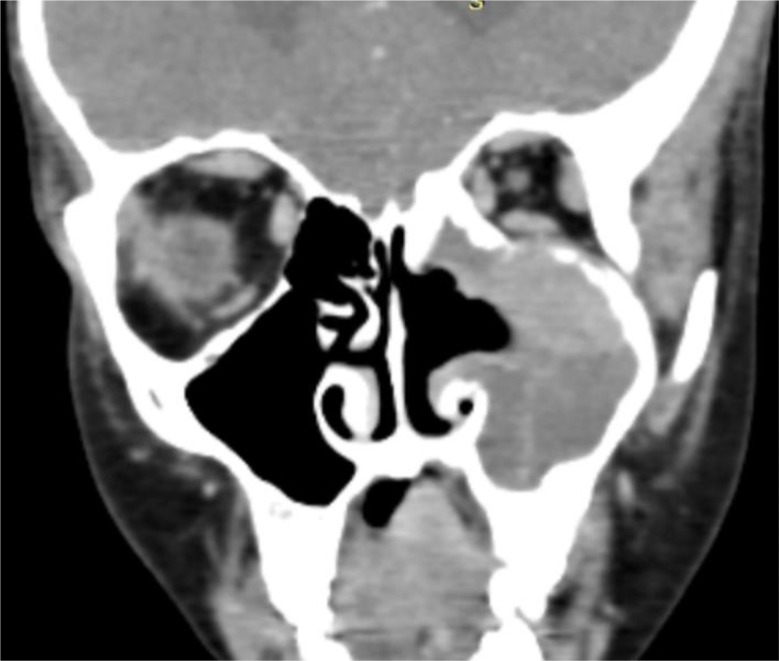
The coronal section of the facial CT scan shows a heterogeneous tissue lesion centered on the left maxillary sinus with lysis of the medial wall and invasion of the lower nasal turbinate.
